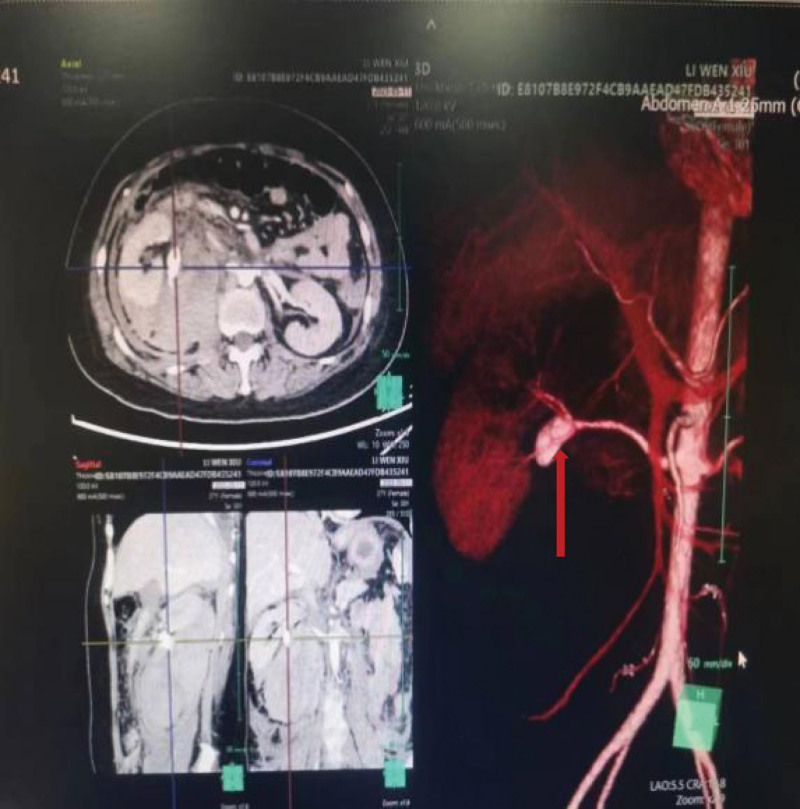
Abdominal enhanced CT + 3D imaging: rupture of the beginning of the inferior trunk branch of the right renal artery and formation of a peripheral hematoma (involving perirenal, retroperitoneal, and pelvic cavity).
